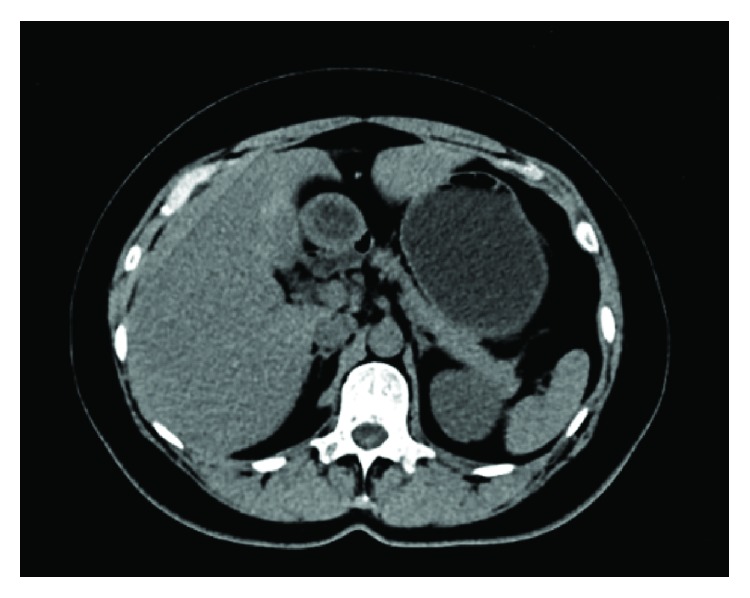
Computed tomography (CT) findings: plain CT scan performed at the diagnosis of cancer showed no hepatic abnormality.
